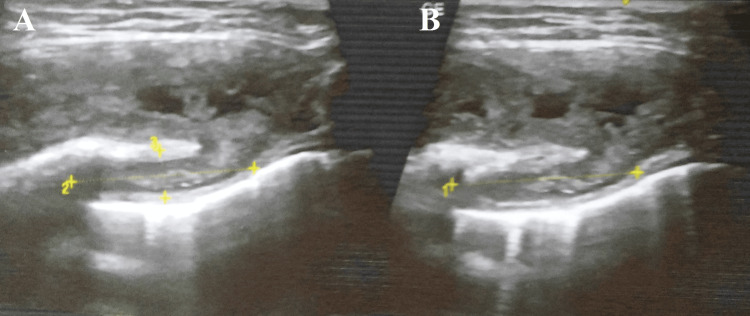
HRUSG showing a collection with deeper intrathoracic extension abutting and compressing anterior lung surface A: Collection with deeper intrathoracic extension. B: Collection showing deeper intrathoracic extension abutting and compressing anterior lung surface. HRUSG - high-resolution ultrasonography
